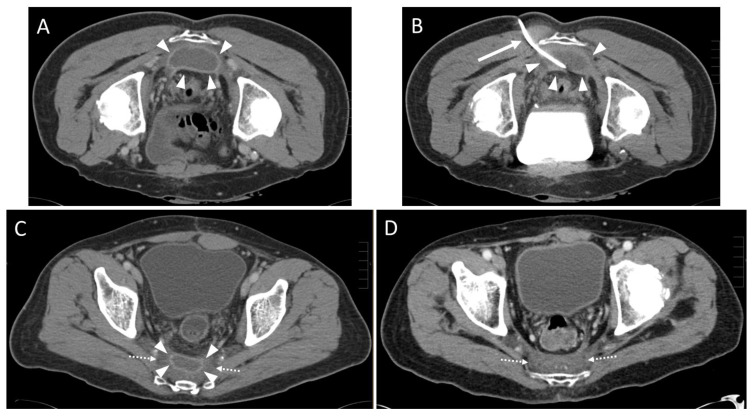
A 59-year-old male with history of rectal cancer (T3 tumor stage) after deep anterior rectal resection including total mesorectal excision and creation of a loop-iliostomy. A control CT scan after 9 weeks revealed a presacral contrast enhancing fluid collection. The patient had no clinical symptoms (Leukocytes: 4.1 × 10^9^/L; CRP: 0.6 mg/dL). (A) Preinterventional contrast enhanced CT scan in prone position showing the presacral fluid collection with rim enhancement (arrowheads). (B) Insertion of an 8 F drain (arrow) into the abscess formation (arrowheads). Only 20 mL of fluid could be aspirated. A microbiological analysis revealed an encapsulated hematoma infected with peptostreptococcus species. (C) Six weeks after the intervention, the fluid accumulation (arrowheads) showed a slight regression in size. Hypodense scar tissue (dotted arrows) had developed. (D) After one year the hematoma was completely resolved. Dotted arrows: scar tissue.
